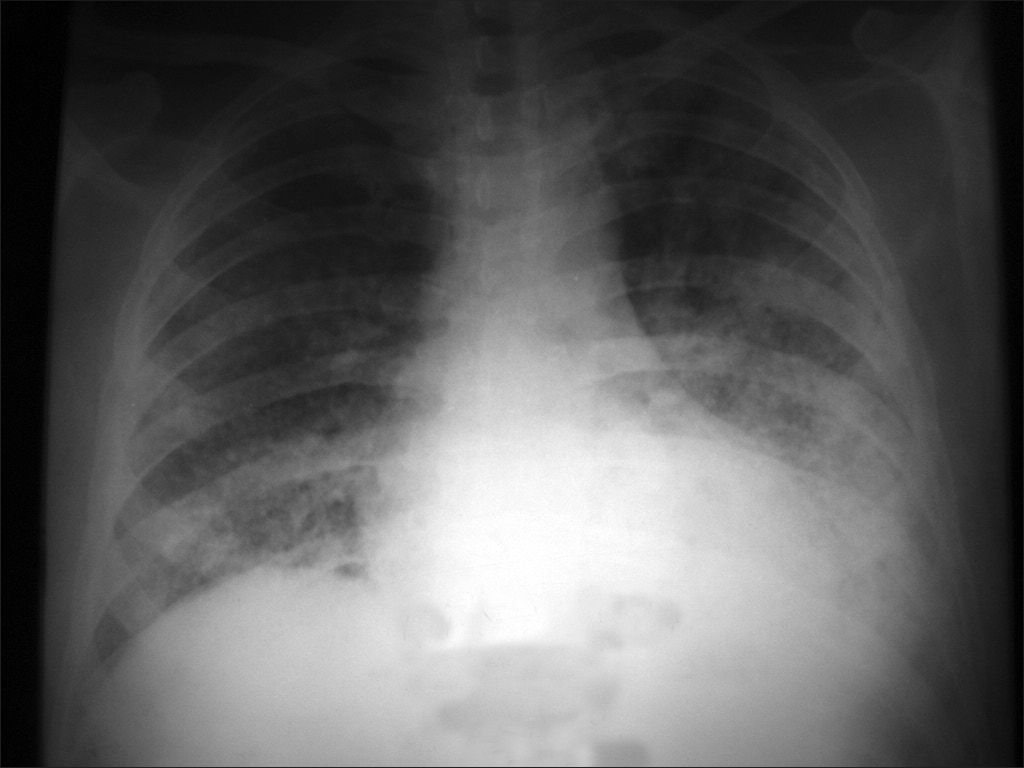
Chest radiograph showing bilateral diffuse alveolar infiltrates
